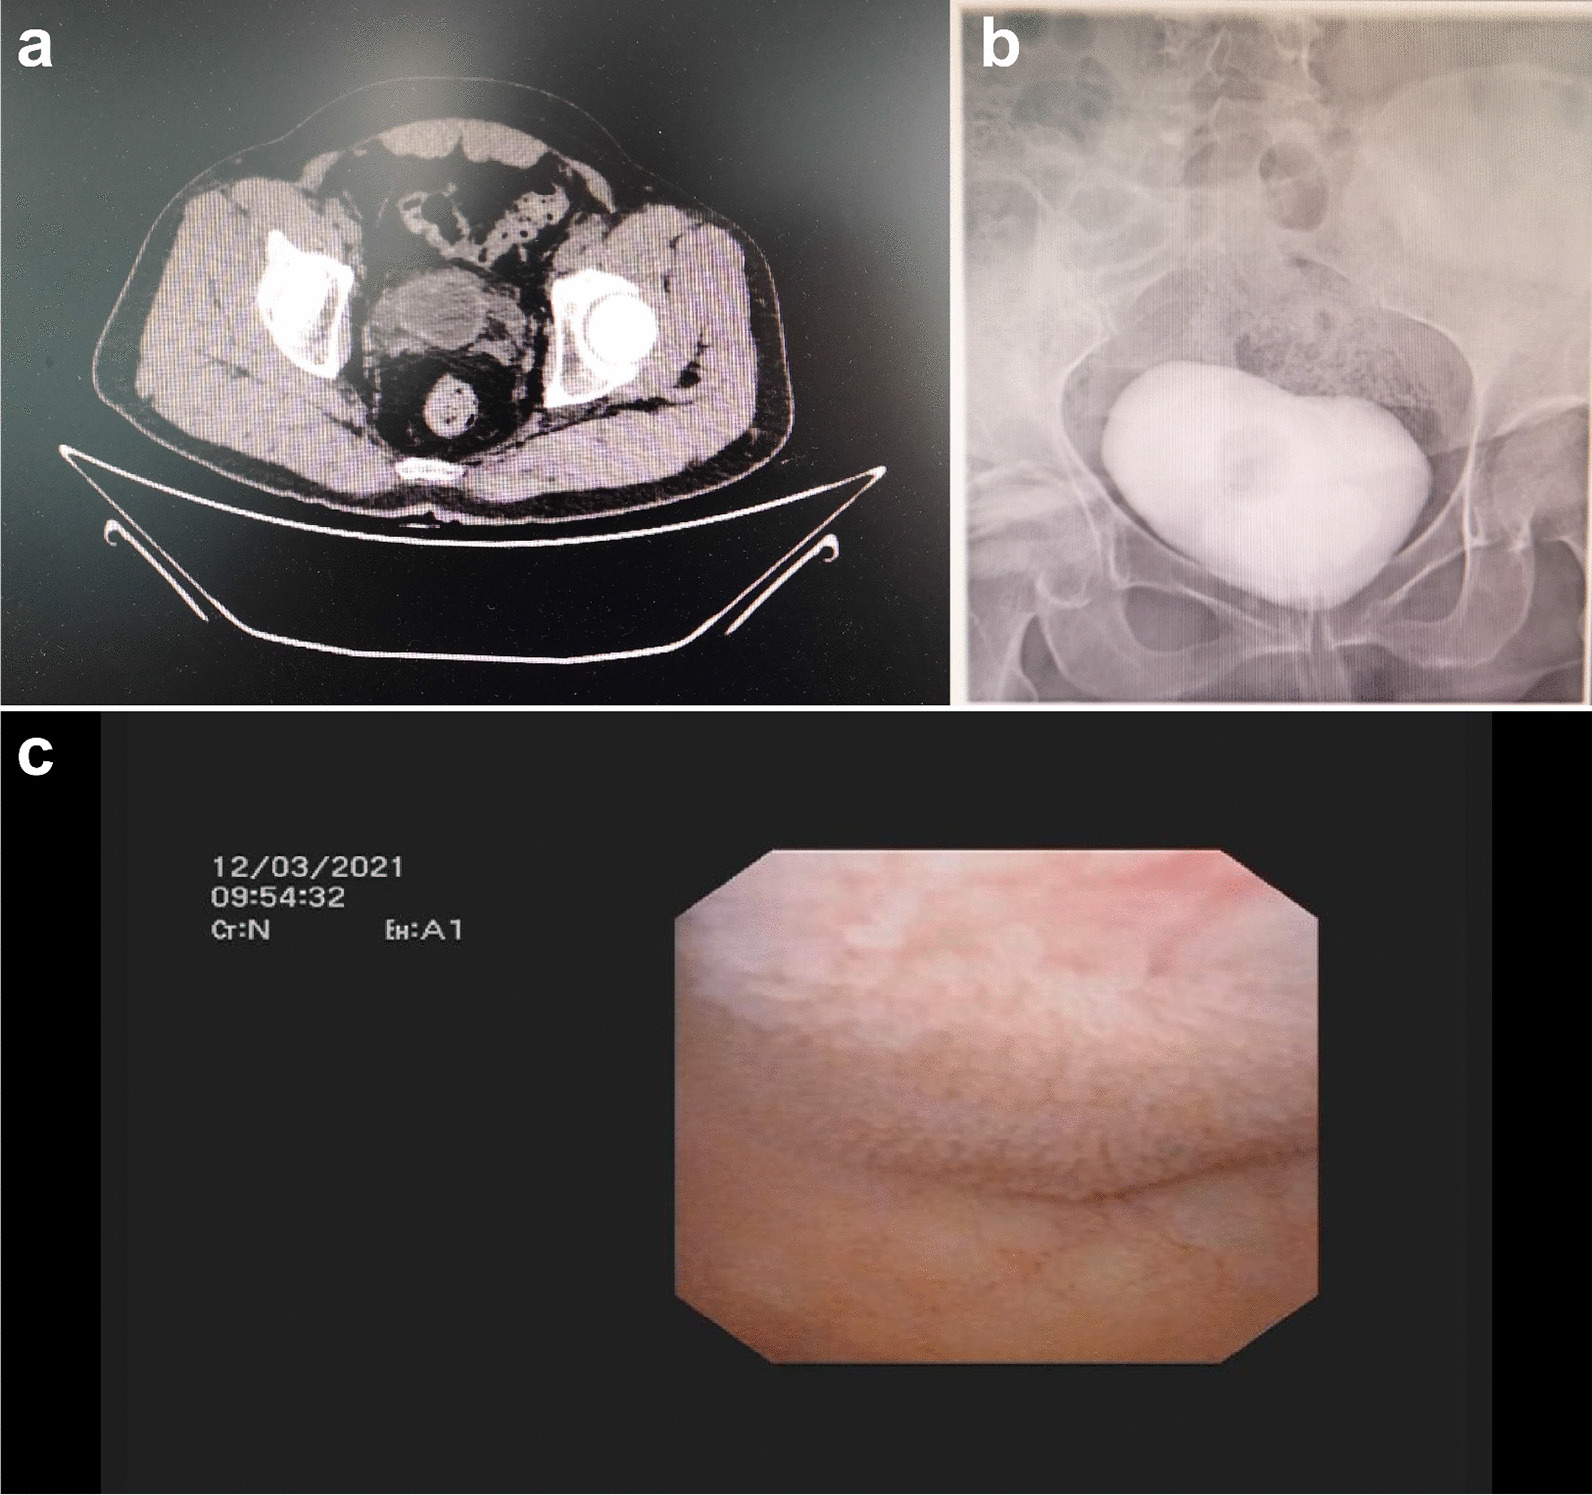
Bladder contracture imaging examination and postoperative cystoscopy results. A pre-op pelvic CT indicated bladder contracture with bilateral ureteral dilation; B cystography at 6 months post-op indicated a significant increase in bladder capacity; C cystoscopy at 6 months post-op revealed a red color in the mucosa of the ileum and bladde wall, with no urolithiasis at the anastomosis
